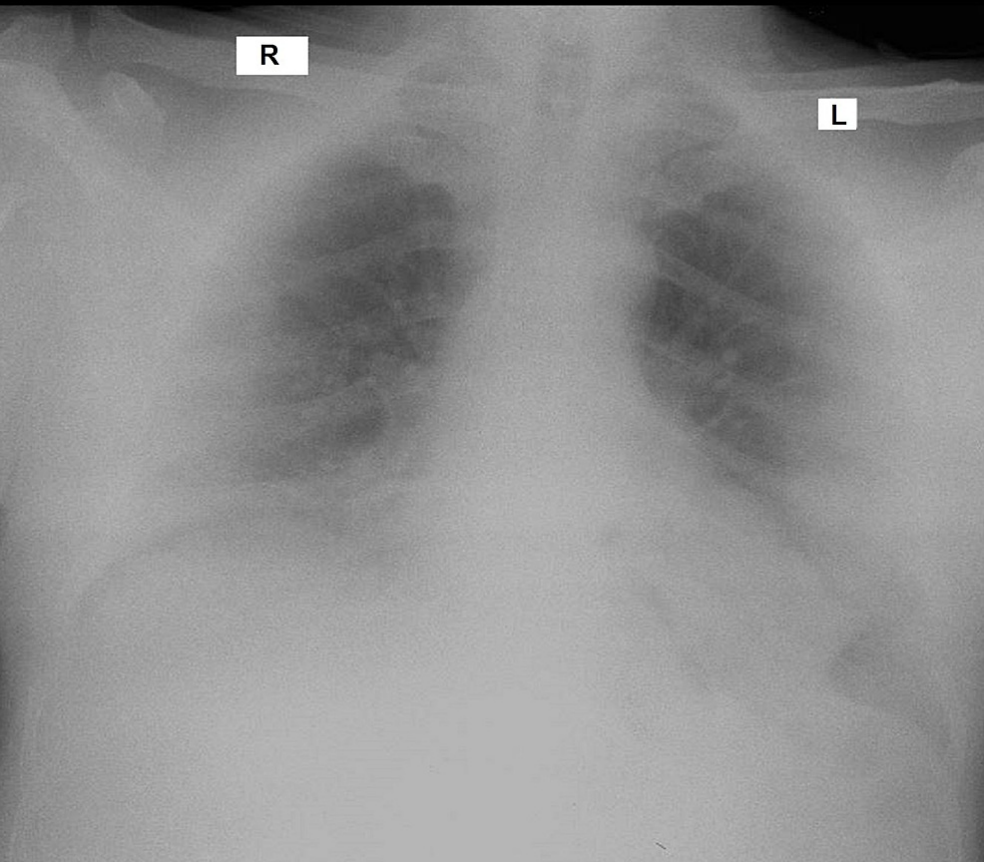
Chest x-ray showing haziness over the bases and periphery.
